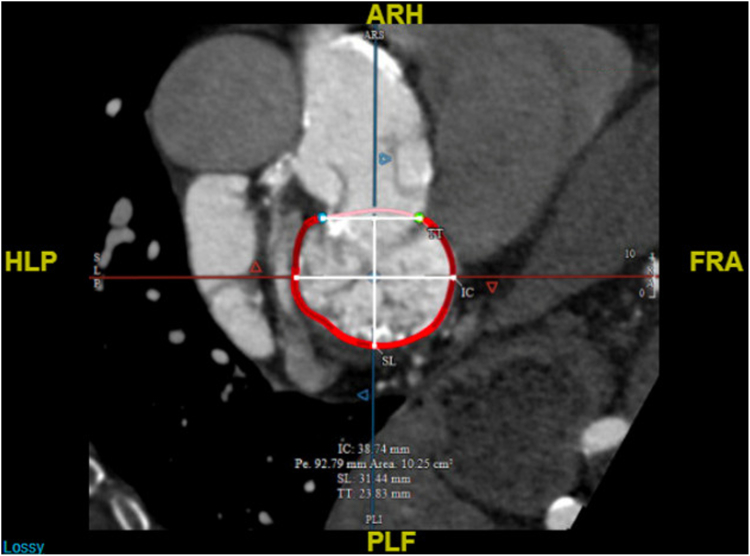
Cardiac CT showing large intercommissural diameter of 38.74 mm for TMVR with ViMAC. Abbreviations: CT, computed tomography; TMVR, transcatheter mitral valve replacement; ViMAC, valve-in-mitral annular calcification.
